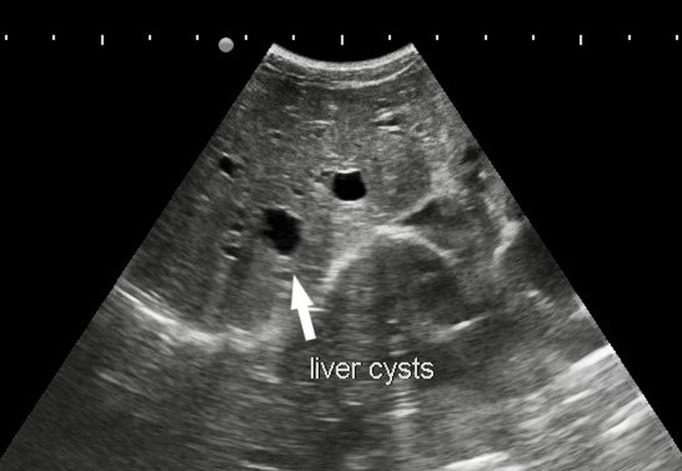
ultrasound sonography test (USG) showing empty renal fossa with non-visualisation of right kidney; multiple varied sized cysts in liver parenchyma
